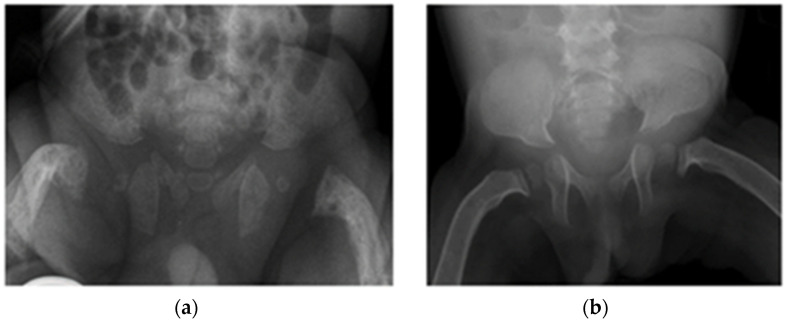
Progression to dysostosis multiplex. Patient 4 (a) Radiograph of pelvis on day 1 shows a reduced bone density and irregularity of the proximal femora with periosteal cloaking. (b) Follow-up radiograph at 2 years old shows normal bone density. The pelvis now has a typical shape of dysostosis multiplex with constriction of the lower part of the iliac bones. There has been interval healing of the rickets of the proximal femora and resolution of the periosteal cloaking.
